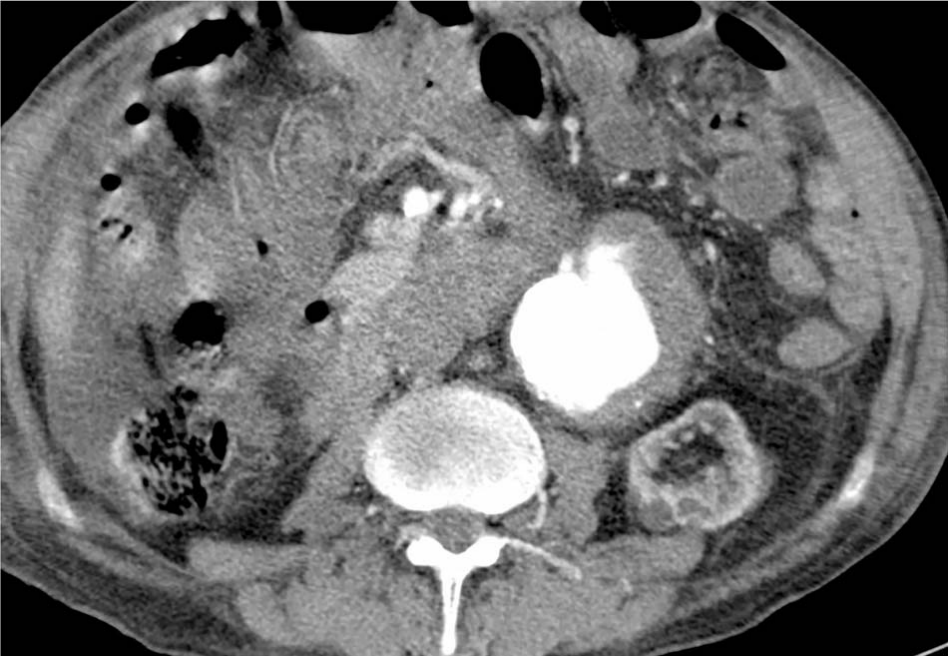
Post-operative contrast-enhanced CT scan, late arterial phase.
